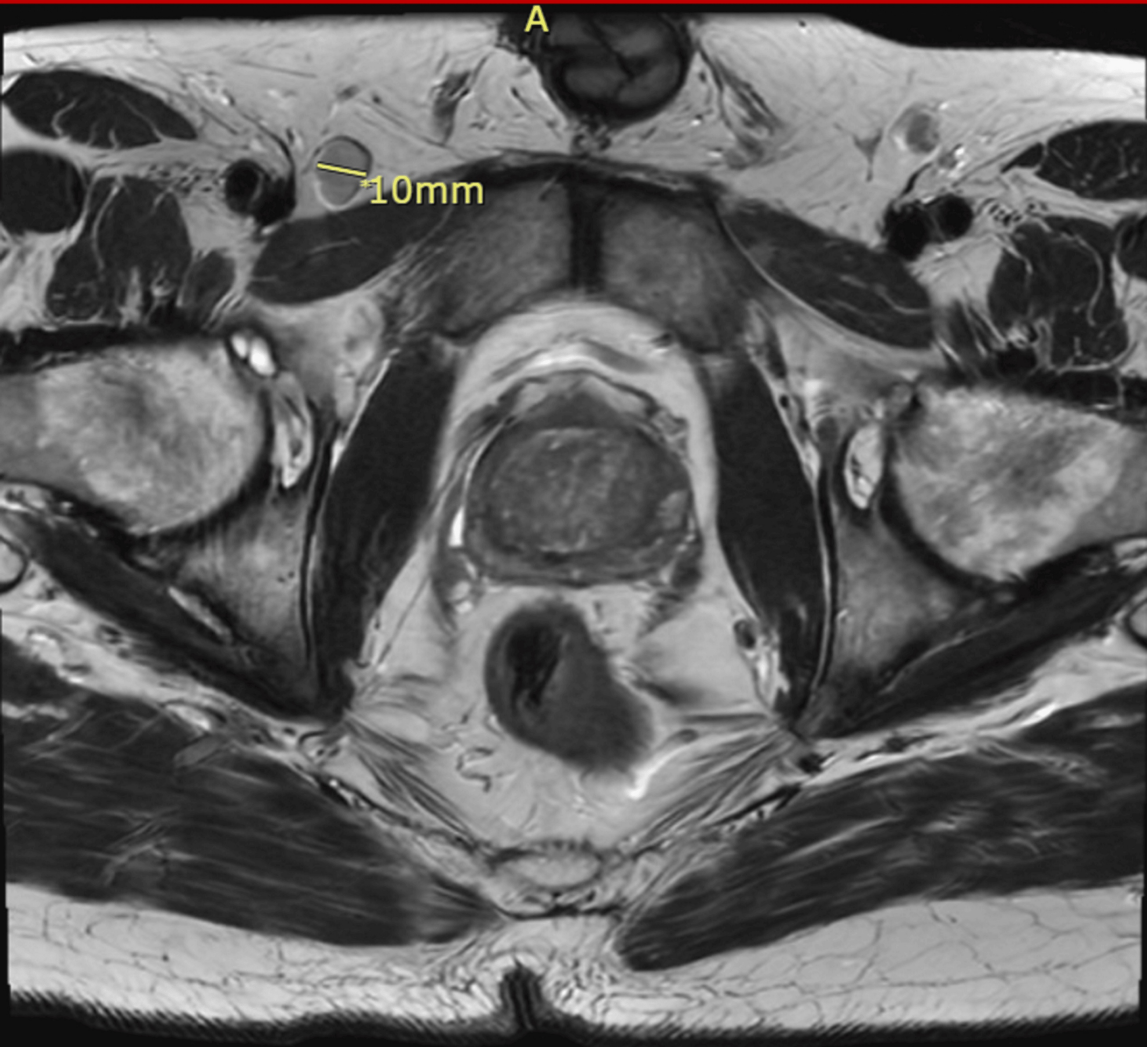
Axial T2-weighted pelvic MRI Axial T2-weighted pelvic MRI demonstrating a 10 mm right inguinal lymph node (label demonstrates short axis diameter), demonstrating concern for regional nodal metastasis in the context of an external perianal lesion. The letter “A” assists with image orientation and indicates the anterior of the patient.
